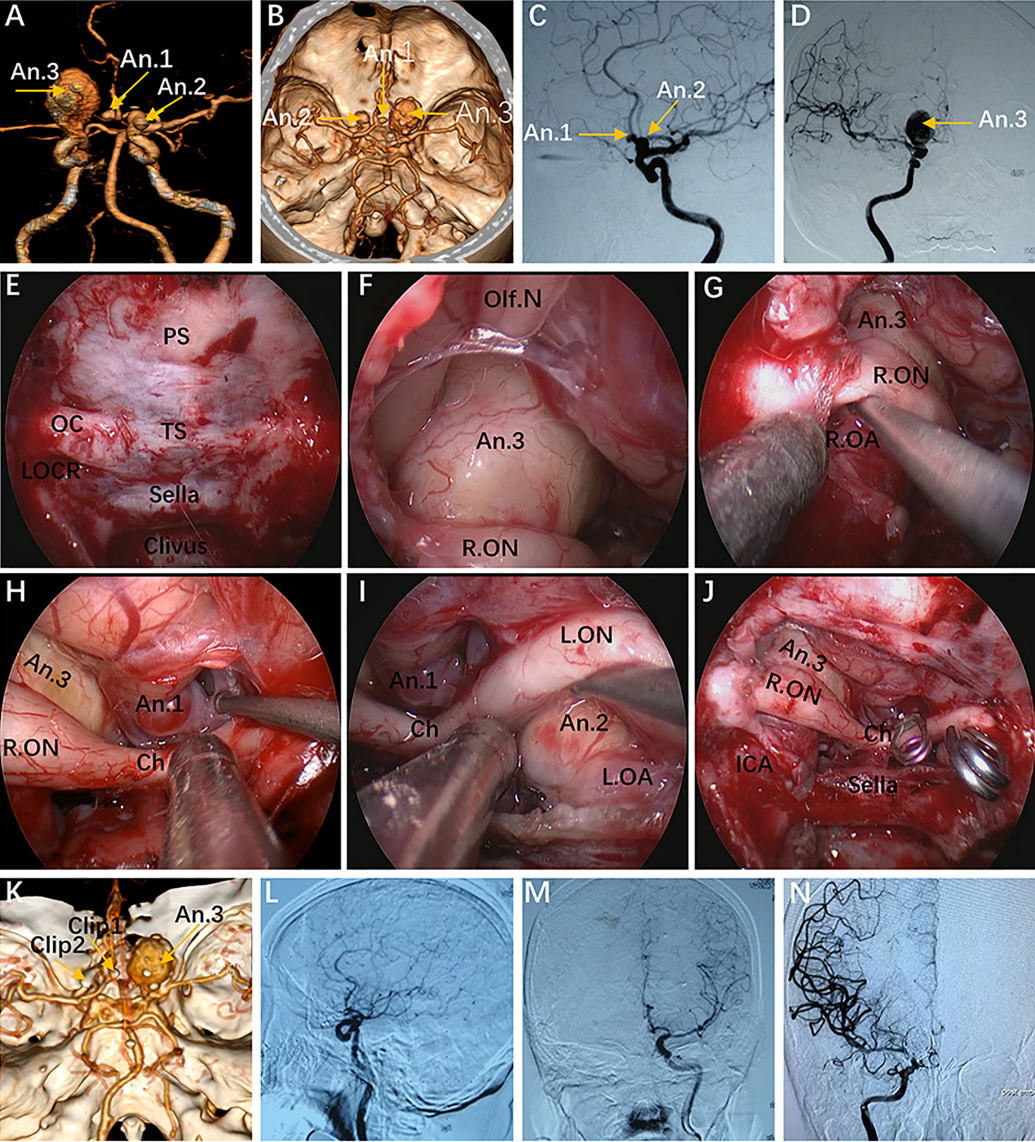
Case 10, a 62-year-old woman, presented with visual loss for 2 months. (A, B) Preoperative CT angiography (A) and a 3-D reconstruction image (B) showing an Acom aneurysm (An1), a left praclinoid aneurysm (An2), and a large right paraclinoid aneurysm (An3). (C, D) Anteroposterior view (C) and lateral view (D) of bilateral internal carotid artery (ICA) injection of digital subtraction angiography (DSA) confirming an Acom aneurysm, a left praclinoid aneurysm, and a large right paraclinoid aneurysm. (E–J) Intraoperative images. (E) Endoscopic view of the skull base showing important bony landmarks. (F, G) Intraoperative image demonstrating an aneurysm body with a thrombus above the right optic nerve that cannot be clipped through an endonasal approach. (H, I) Exposure to the Acom aneurysm (H) and left paraclinoid aneurysm (I). (J) Intraoperative picture showing that both aneurysms were clipped successfully after proximal control. (K) Postoperative CT angiography confirmed the patency of the bilateral A2 and distal ICA. (L, M) Postoperative lateral view (L) and anteroposterior view (M) of left ICA injection of DSA, revealing complete obliteration of An1 and An2. (N) Postoperative anteroposterior view of right ICA injection of DSA, showing complete obliteration of the An3 after second-stage coiling. An, aneurysm; R.OA, right ophthalmic artery; L.OA, left ophthalmic artery; Ch, optic chiasm. The figure is available in color only online.
